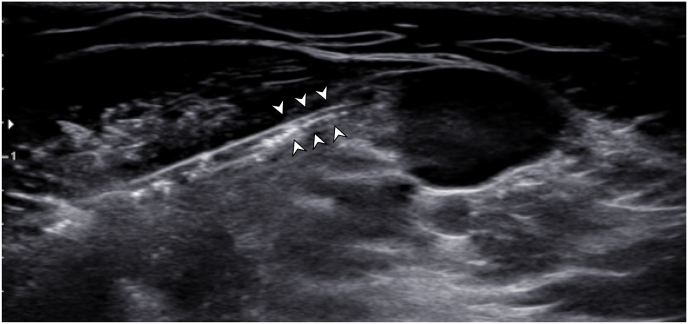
Ultrasound image displays the introducer needle approaching the region between the median nerve and ulnar nerve.
